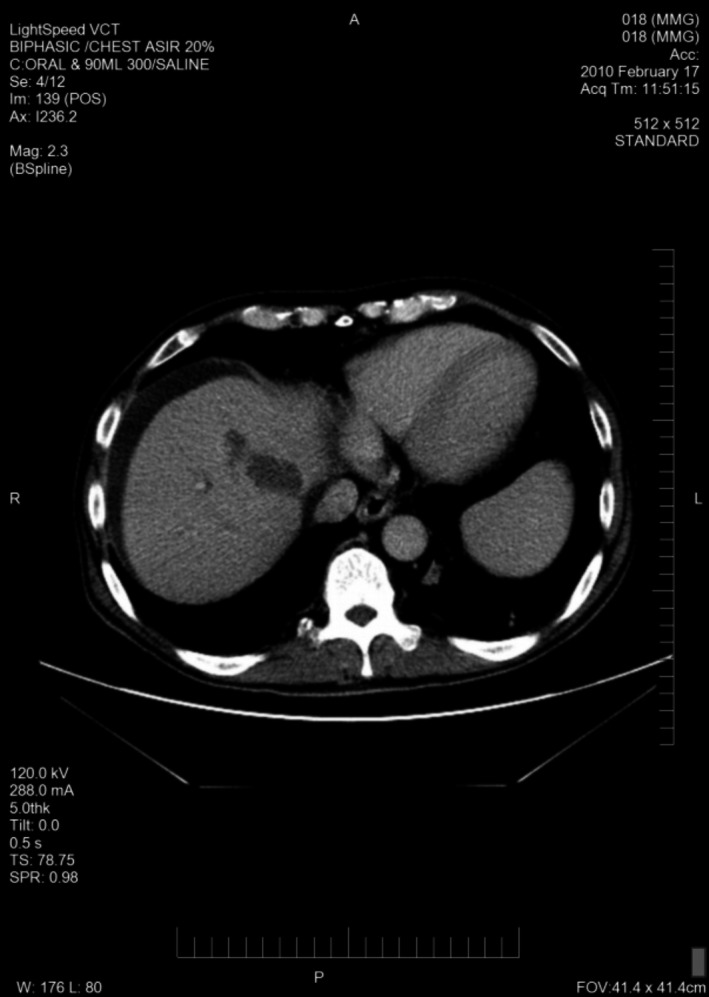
Patient 1 with Hepatocellular Carcinoma (HCC), 6‐month follow‐up CT portal venous phase.
